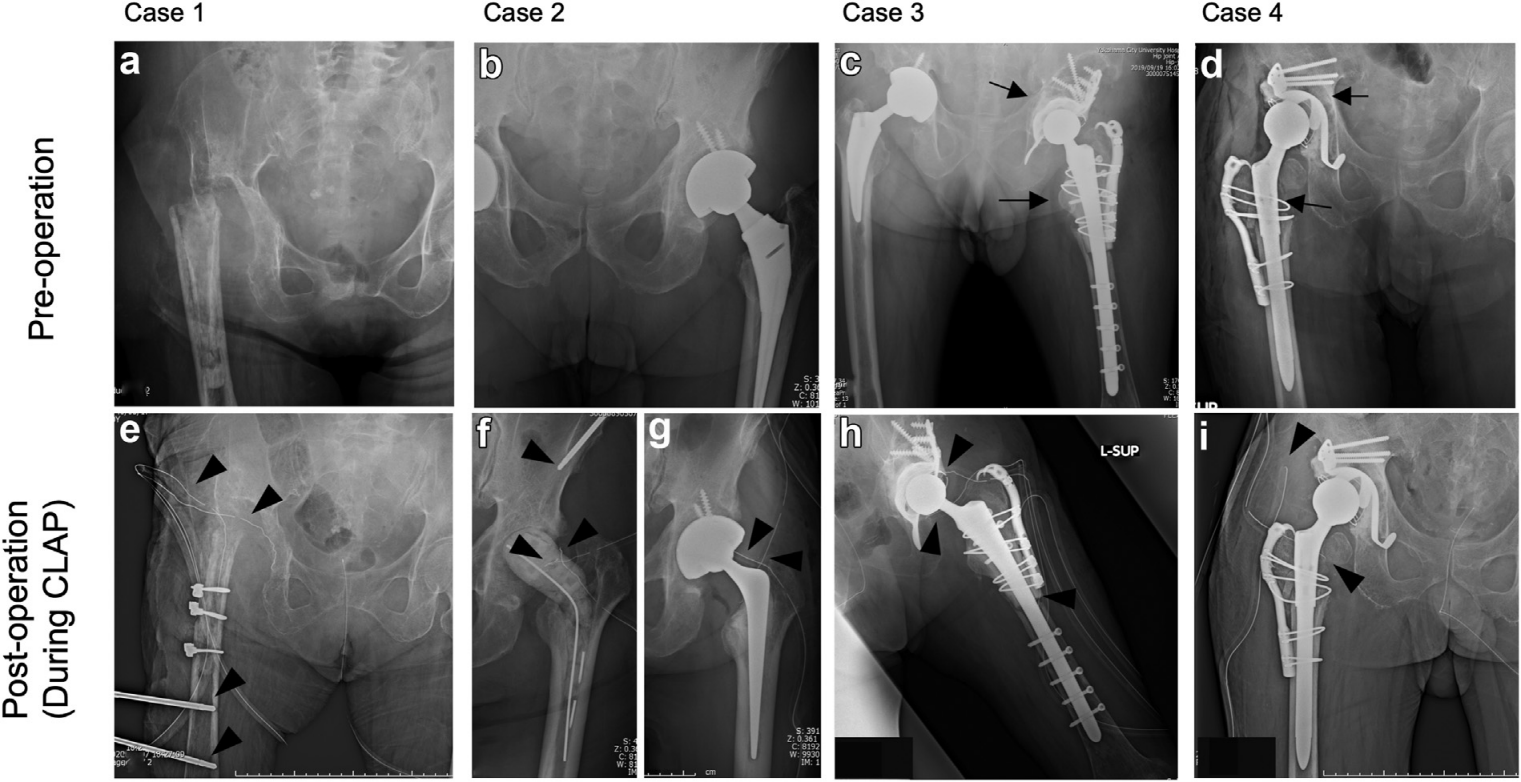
Preoperative and postoperative radiography in fungal periprosthetic joint infections (PJI). (a-d) Preoperative radiography for fungal PJI cases including patients with resection arthroplasty with massive bone defects (a, case 1), septic implant loosening (b, case 2), and patients after revision total hip arthroplasty (THA) with a support cage and cable plate with allogeneic bone grafts (arrows in c and d, cases 3 and 4). (e-i) Salem Sump Tubes were used in combination with iMap pins in femur and acetabulum (arrow head in e and f) to perfuse micafungin to infected site in debridement (e, case 1), 2-stage revision THA (f, case 2), and implant retention (h and i, cases 3 and 4) cases.
